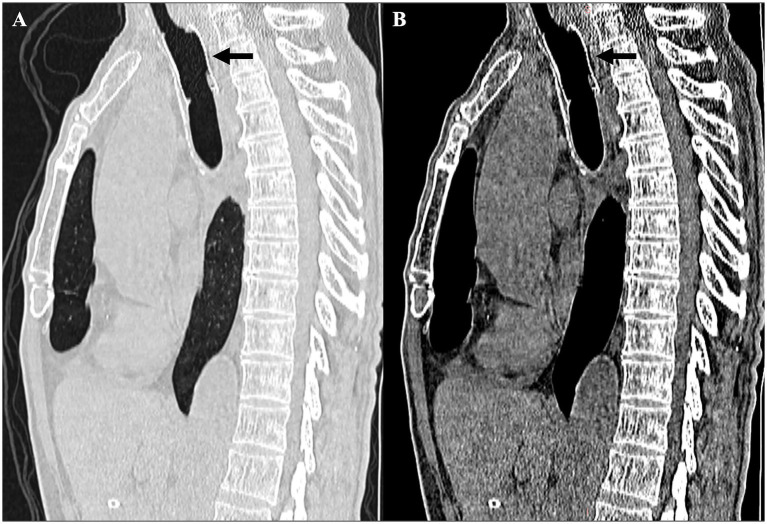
Serial chest computed tomography (CT) imaging after the procedure. (A,B) Sagittal CT scans (lung and mediastinal windows): stent shadow seen in cervical trachea (black arrow), with obliteration of periesophageal fat planes. Tracheal patency was improved compared with the previous imaging. The previously noted air-containing cavity had disappeared (black arrowhead), indicating no definite tracheoesophageal fistula.
